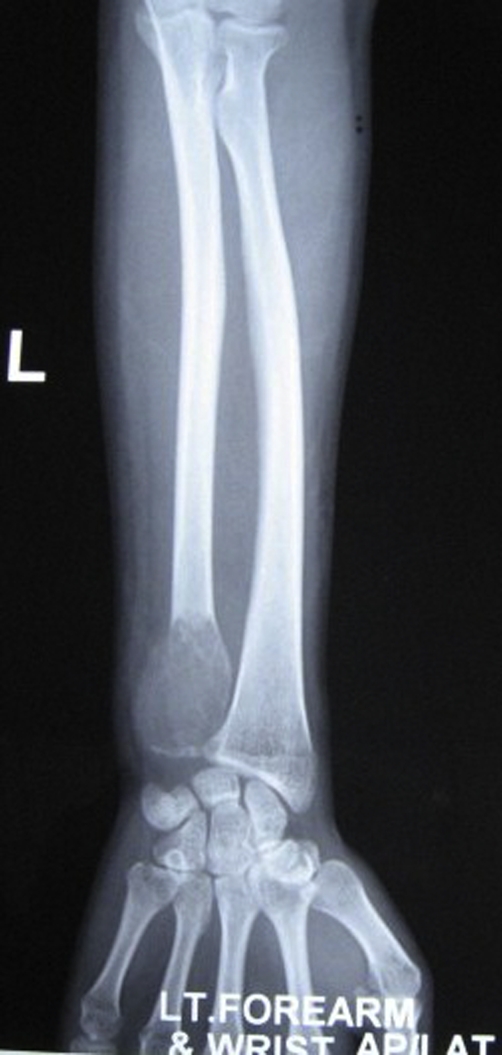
Case #2. AP Radiograph of left forearm showing a lytic lesion of the distal aspect of ulna with an indistinct medial margin and absence of periosteal reaction.
